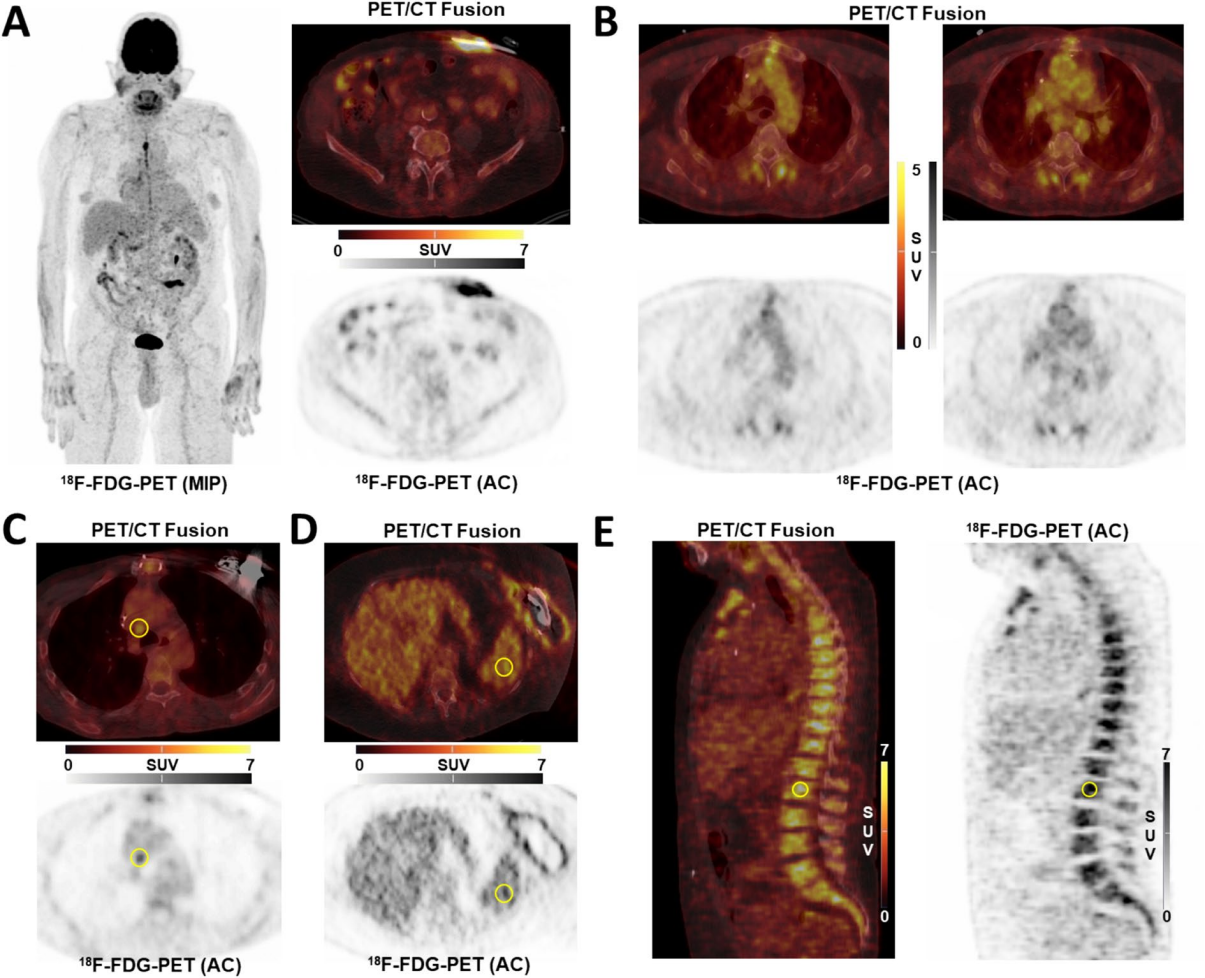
(A) Infection of driveline entry site; (B) Thoracic vessel signal; (C) Mediastinal lymph node signal; (D) Spleen signal; (E) Bone marrow signal; *AC* attenuation-corrected image; ^18^*F-FDG*
